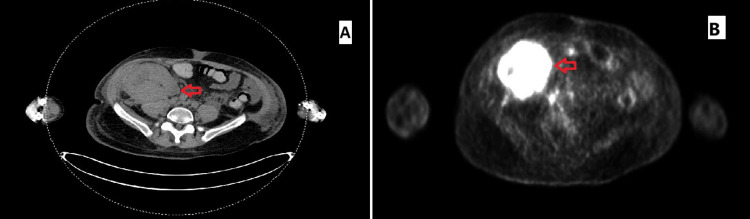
Case 2: PET-CT (A) The transplanted kidney is enlarged, indicating biopsy-proven KS. (B) The imaging shows intense, inhomogeneous uptake. KS, Kaposi sarcoma
